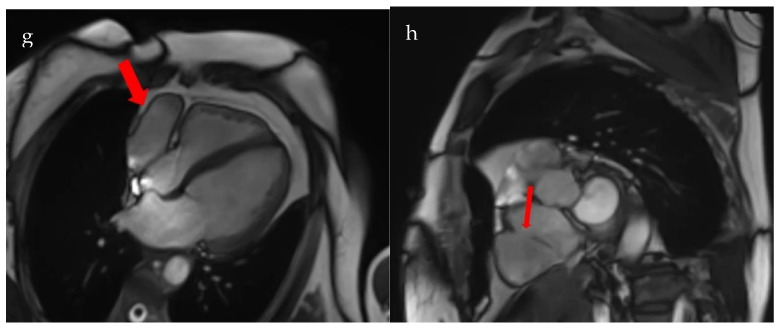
Cardiac MRI. (a). Diastolic view; (b). Systolic view; (c). Early T1 acquisition; (d). T2 acquisition; (e,f). LGE sequences: mid-myocardial contrast uptake with non-ischemic pattern at the interventricular septum and right ventricular insertion junction; (g,h). Right atrium diverticulum (red arrow).
